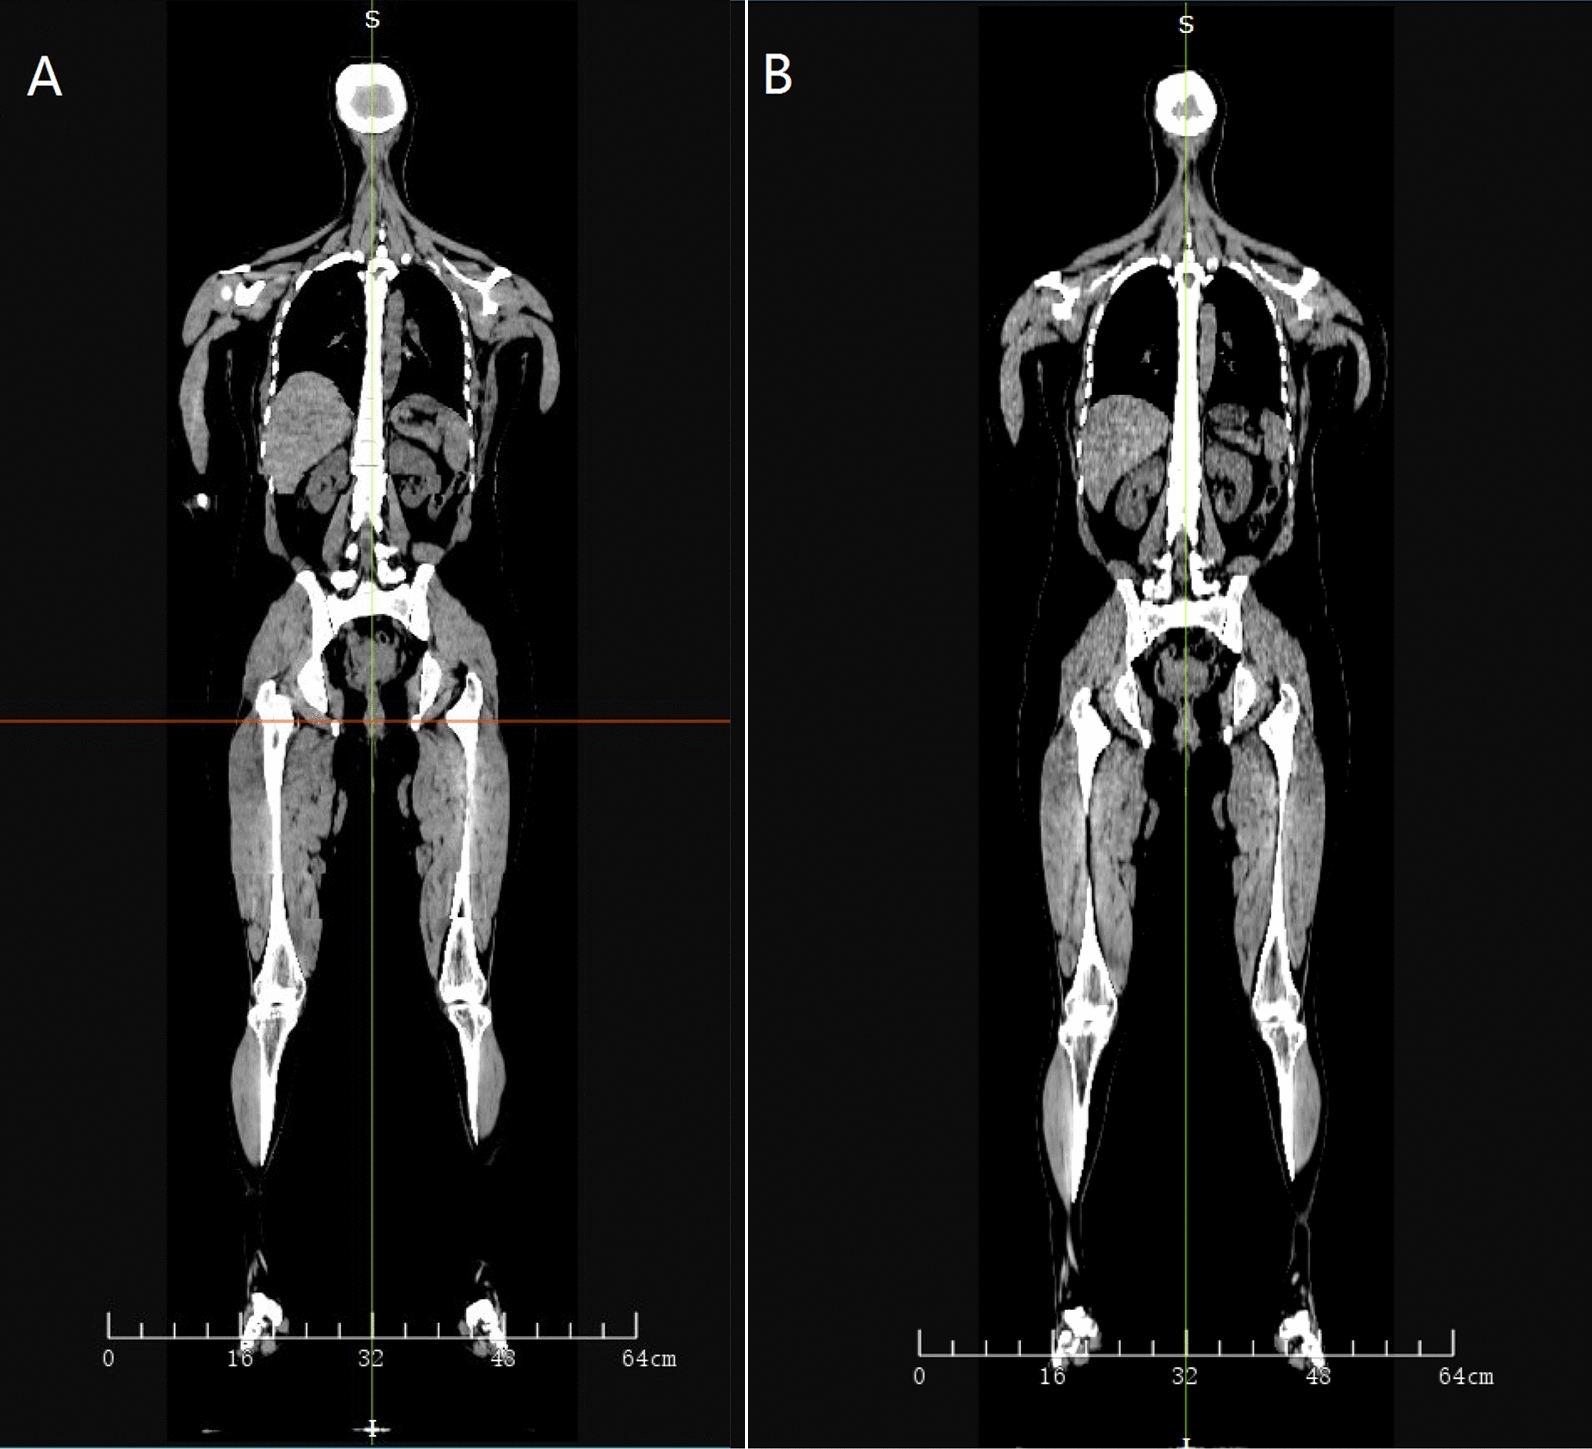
Examples of the whole body CT. A Planning CT. B FBCT scanned in the first fraction
